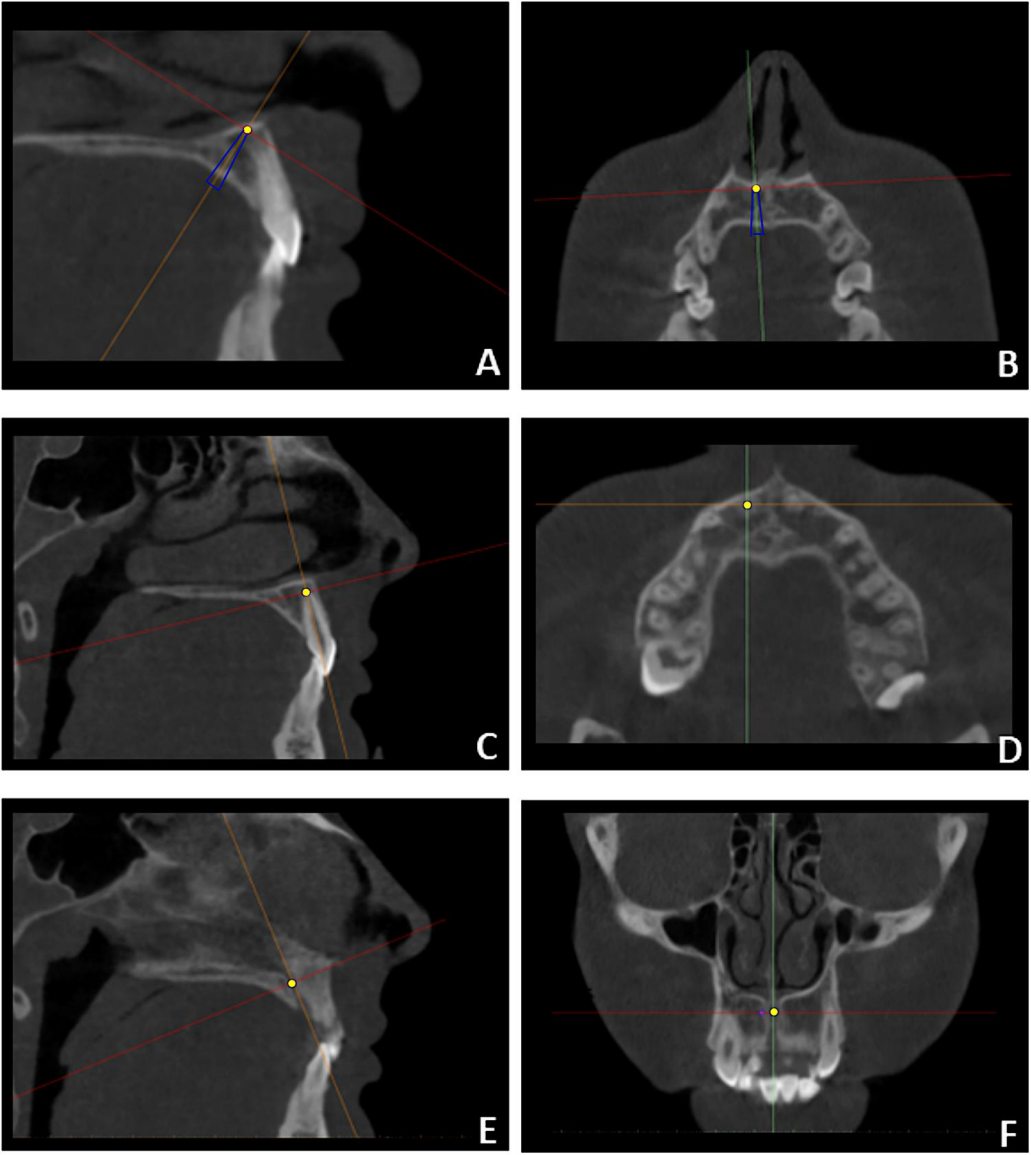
CBCT orientation and identification of landmarks used for the analysis: miniscrew's tip (A,B), incisor's apex (C,D) and the minimum distance between the miniscrew and the nasopalatine duct (NP) (E,F). These landmarks were retrieved after orientation of the *y*-axis respectively along central axis of the miniscrew (A,B), the central axis of each maxillary incisor (C,D) and the axis of the nasopalatine duct (E,F).
